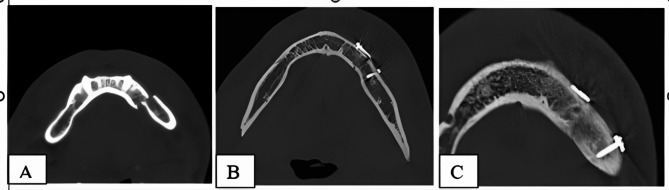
A. Preoperative CT axial cuts. (B) Immediate postoperative CT axial cuts. (C) 3 months postoperative axial cuts showing fixation and healing of the fracture line
